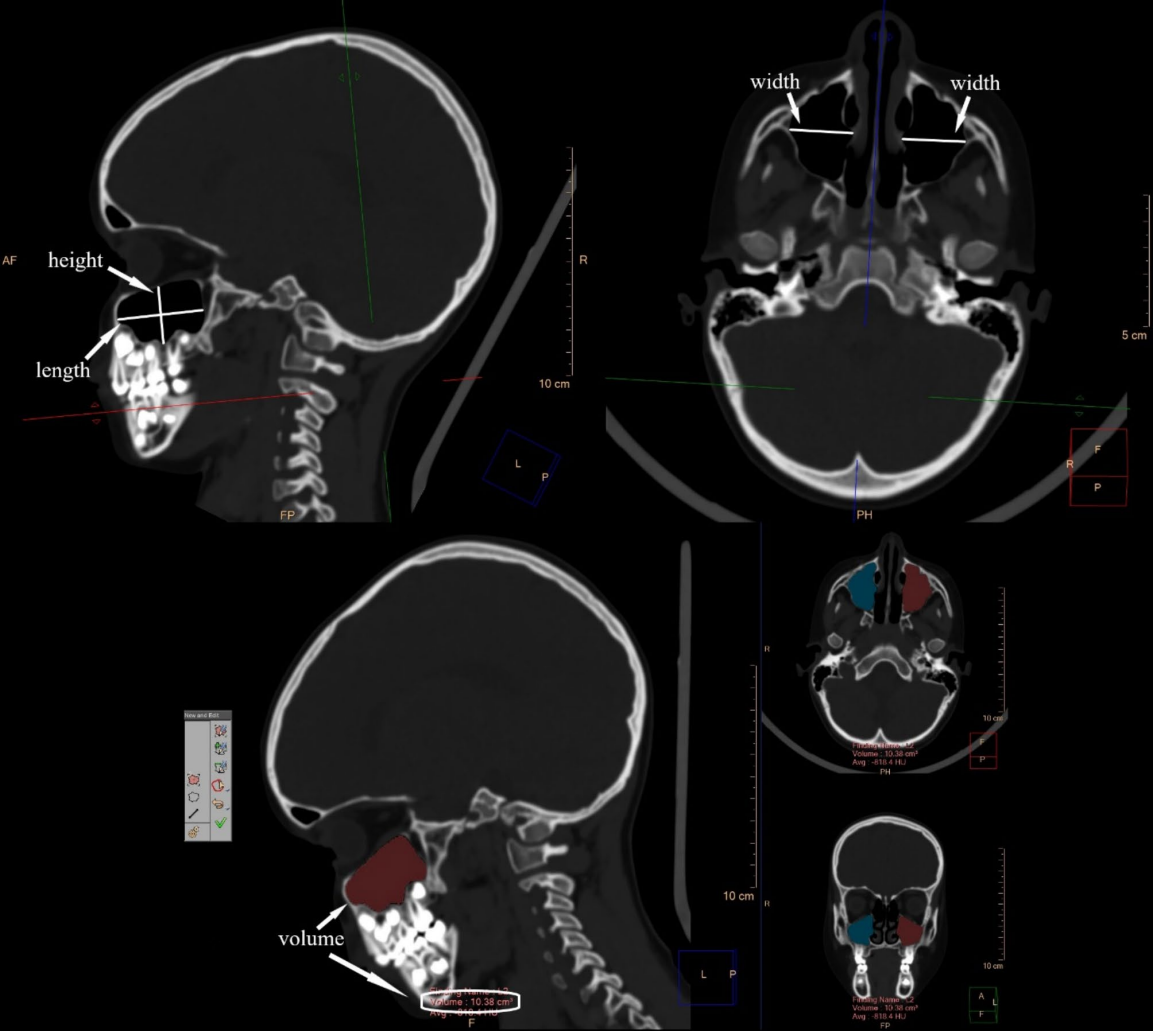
Computed tomography of the head in children showing the measurements of the maxillary sinuses: height, length, width and volume used in the study.
